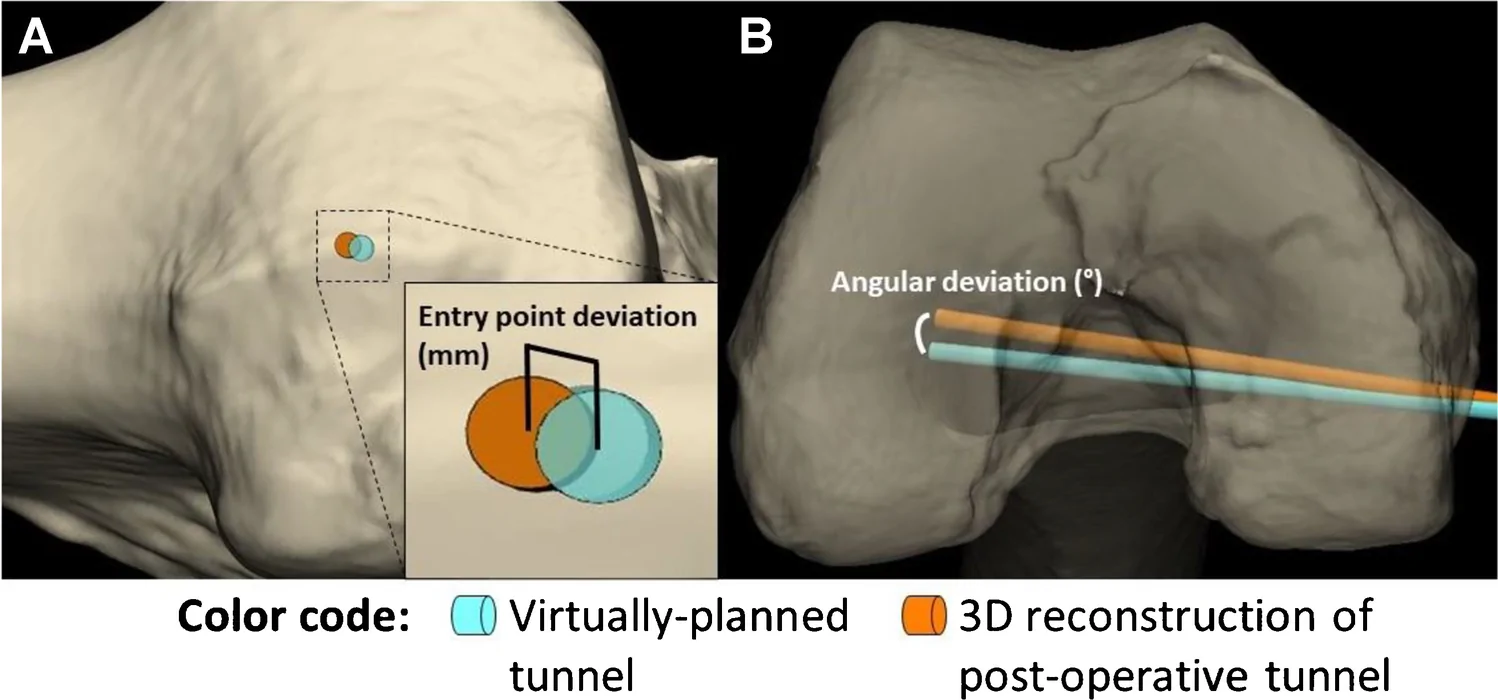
A–B Superimposition of 3D reconstruction of pre- and post-operative CT scans. Measurements of A entry point deviation and B angular deviation between planned (blue) and actual (orange) tunnels
